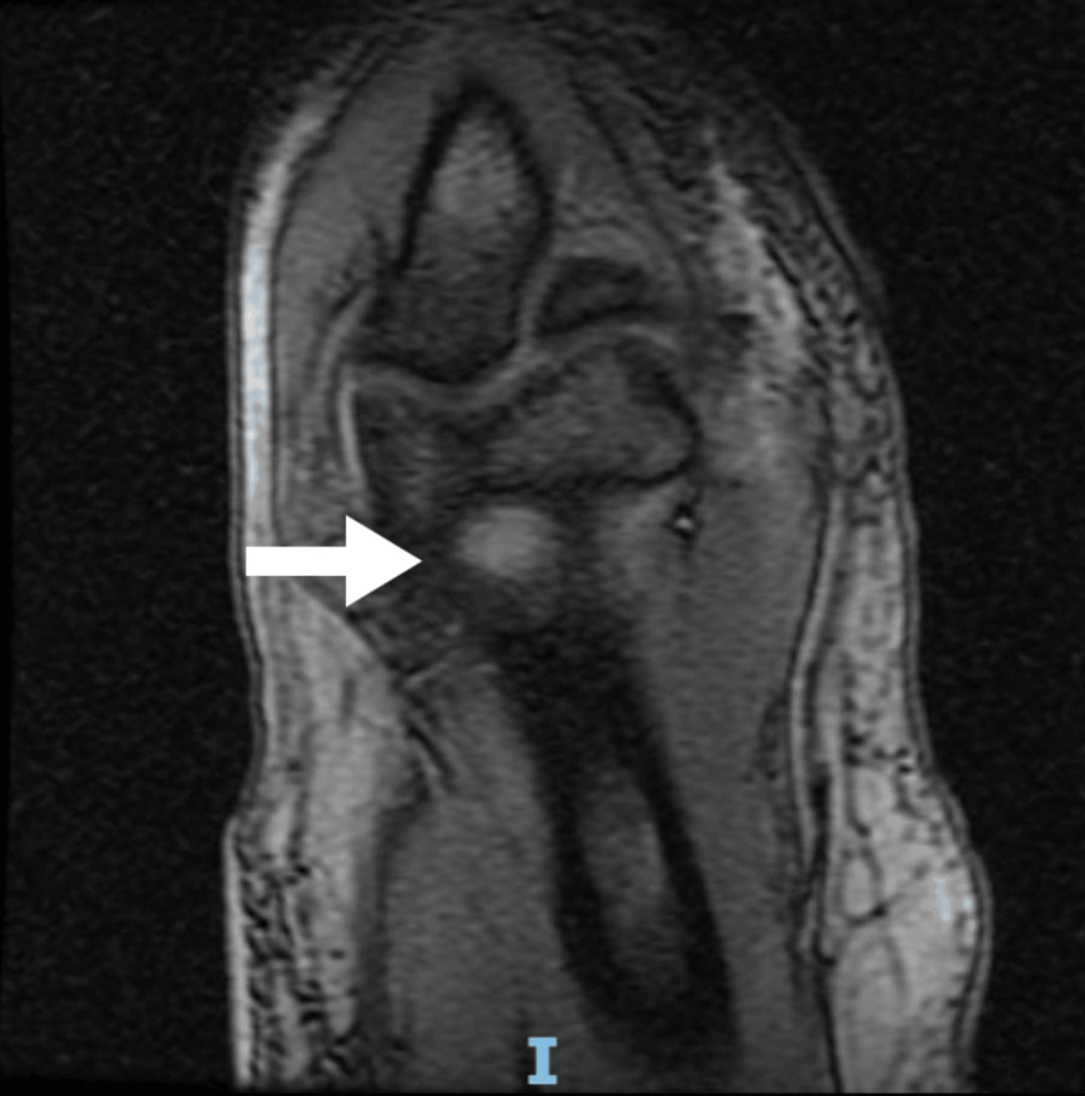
Left elbow MRI. The arrow indicates the location of the capitellum with bone marrow edema and changes suggestive of osteomyelitis.
